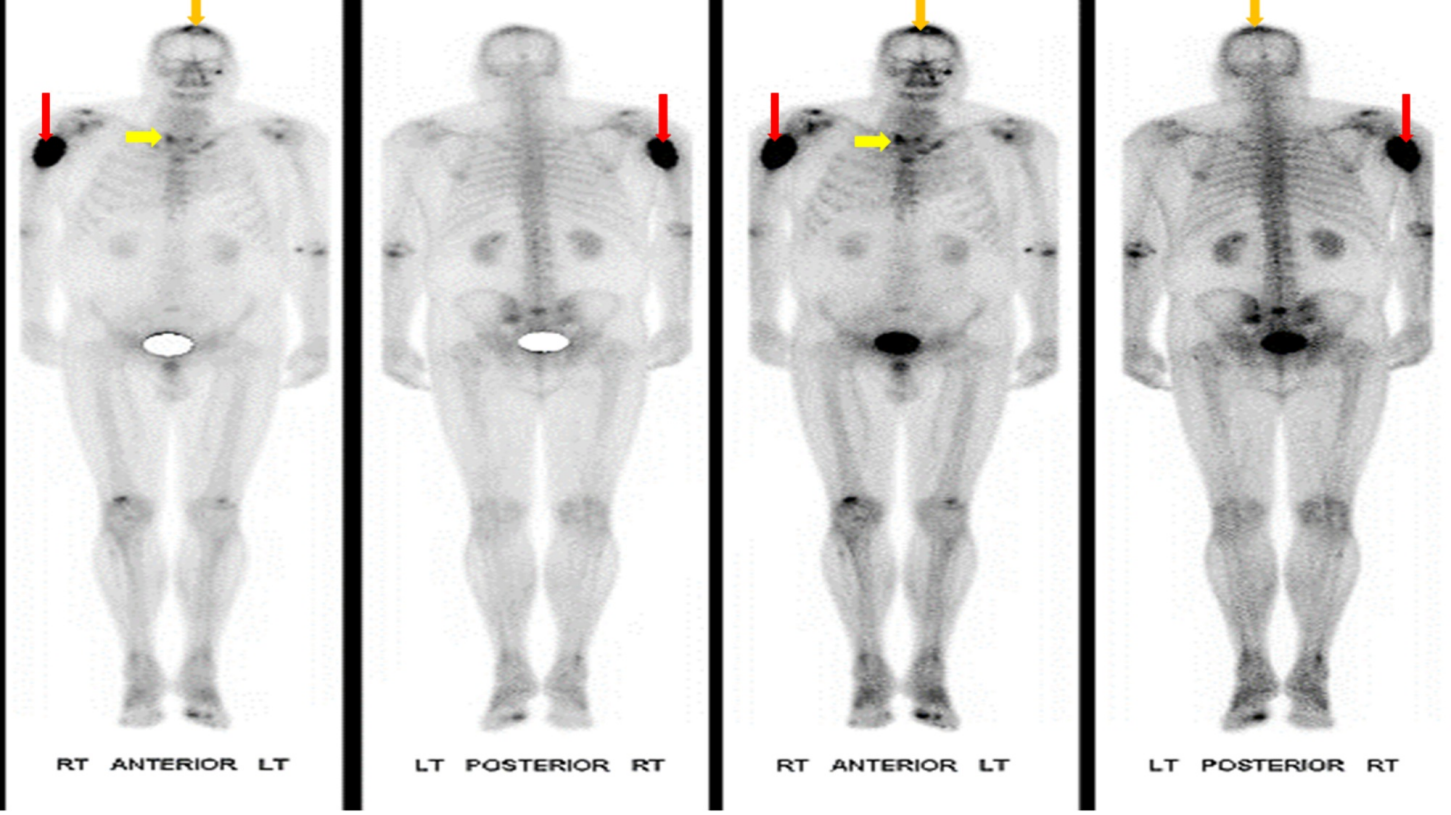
Bone scintigraphy of the patient, showing an increased uptake in the right proximal humerus (red arrows) as well as the apex of the skull (orange arrows). There is also an increased uptake in thyroid gland (Yellow arrows).
